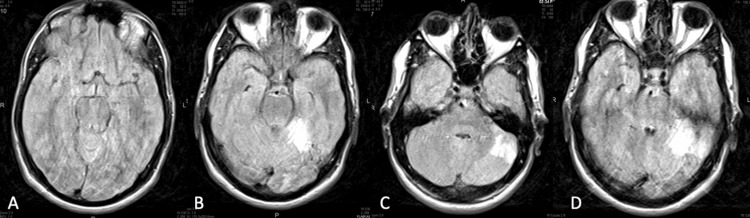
Magnetic resonance imaging/Magnetic resonance angiography. Bilateral inferior cerebellar hemisphere (posterior inferior cerebellar artery territories) patchy acute and subacute infarctions, possible right medulla subacute infarction, left superolateral cerebellar hemisphere subacute infarction, right thalamus medial focal acute infarction, and absent right vertebral artery flow void, suggesting either occlusion or reduced flow.
